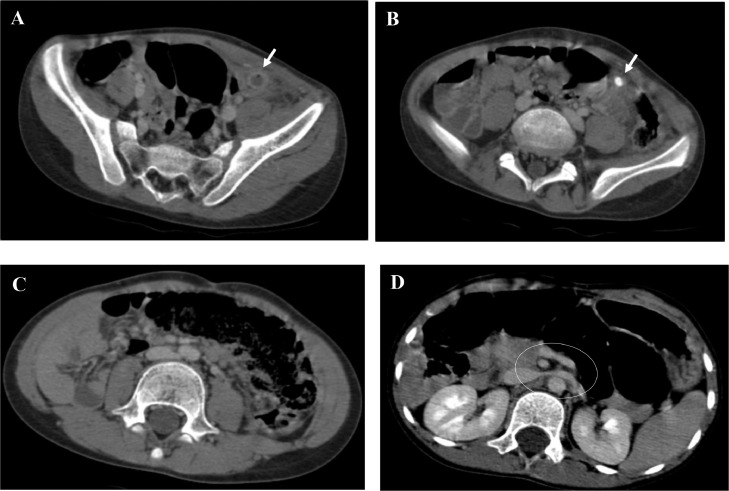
Axial abdominal CT scan (A-B-C) showing: (A) Dilated structure in the left iliac fossa with enhanced mucosa consistent with acute appendicitis (White arrow). (B) Left-sided appendicitis with appendicolith (White arrow). (C) Small bowel on the right and large bowel on the left. (D) Abnormal course of D3 which does not cross the midline (white circle).
